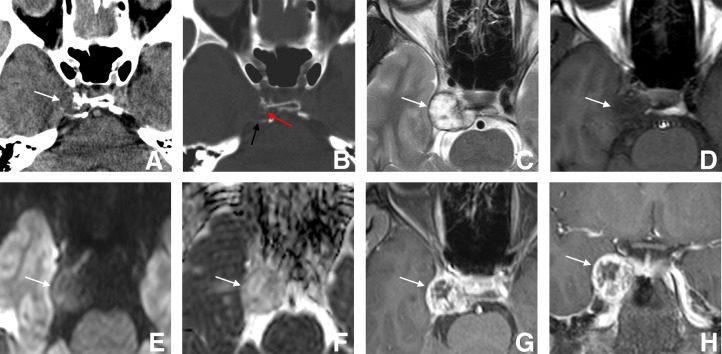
Radiological findings. (A) Axial unenhanced CT (soft tissue window) showing a low-density mass (white arrow) centered on the right clivus and extending into the right cavernous sinus with adjacent osseous destruction. (B) Axial unenhanced CT (bone window) showing central (black arrow) and peripheral (red arrow) linear calcifications. (C) Axial T2-weighted image showing a markedly hyperintense mass (white arrow). (D) Axial T1-weighted image showing a hypointense mass (white arrow). (E) Axial diffusion-weighted image (b = 1000 s/mm²) showing a hypointense mass (white arrow). (F) Axial apparent diffusion coefficient (ADC) map showing a high ADC value (1.96 × 10⁻³ mm²/s) (white arrow). (G, H) Axial (G) and coronal (H) fat-suppressed contrast-enhanced T1-weighted images showing heterogeneous and predominantly peripheral enhancement (white arrow).
